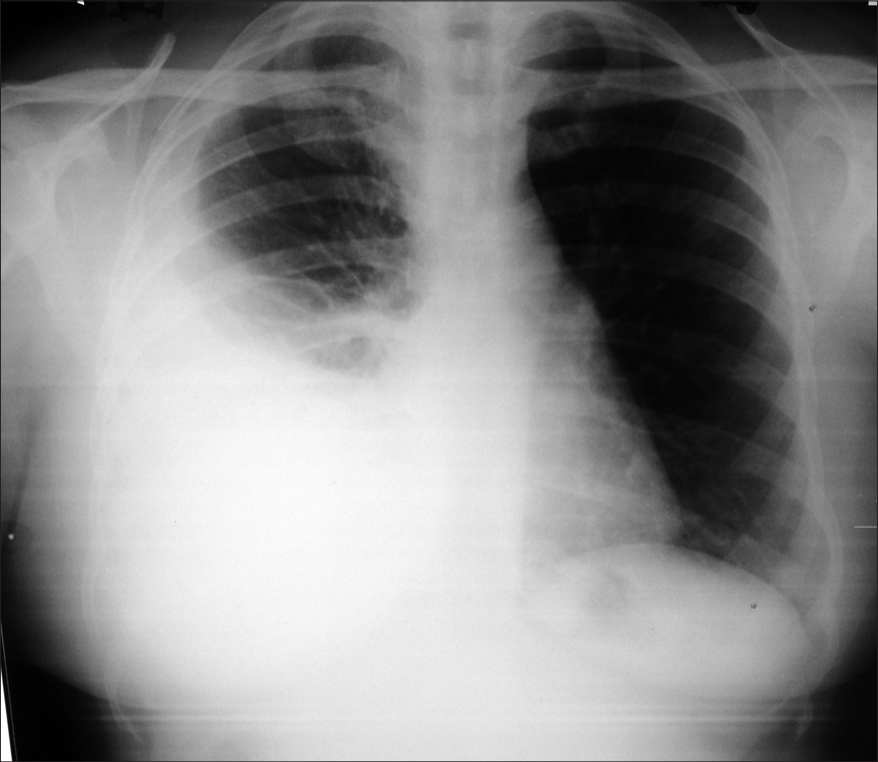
Chest X-ray of patient showing pleural effusion
